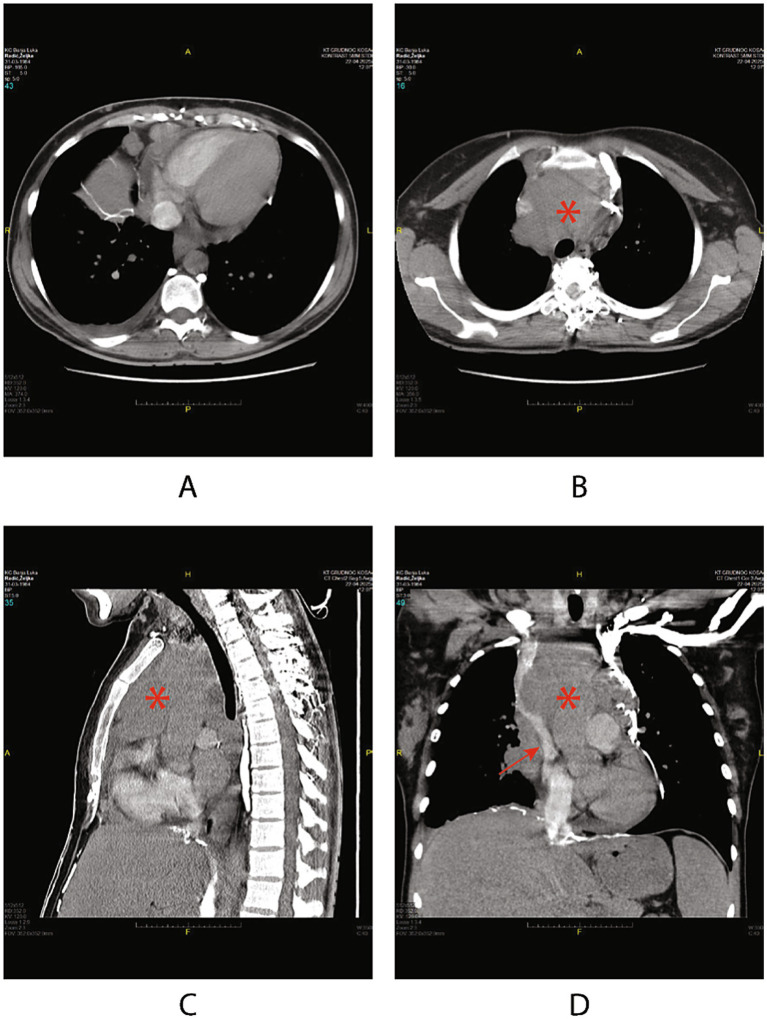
Contrast-enhanced chest CT images demonstrating superior vena cava syndrome. Panels (A, B) show axial sections, and panels (C, D) show sagittal reconstructions. CT of the thorax demonstrating a bulky mediastinal mass (*) causing extrinsic compression of the superior vena cava (arrow), consistent with superior vena cava syndrome.
